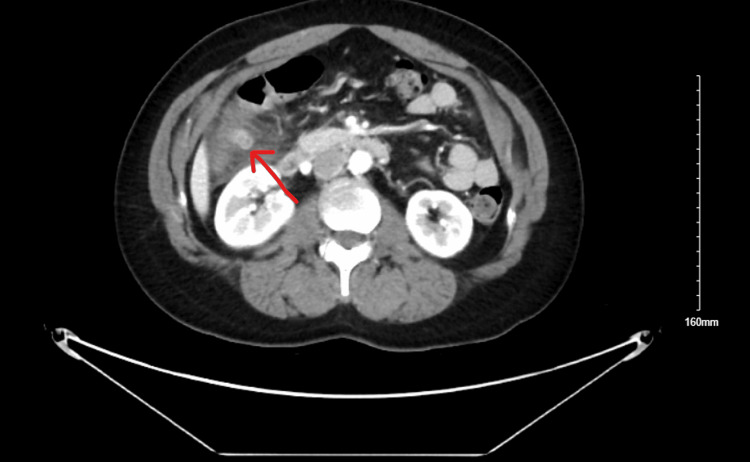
Abdominal CT showing a 14 mm oval, ill-defined soft tissue density near the hepatic flexure with adjacent inflammatory changes in the fat consistent with epiploic appendagitis (red arrow) CT: Computed tomography
